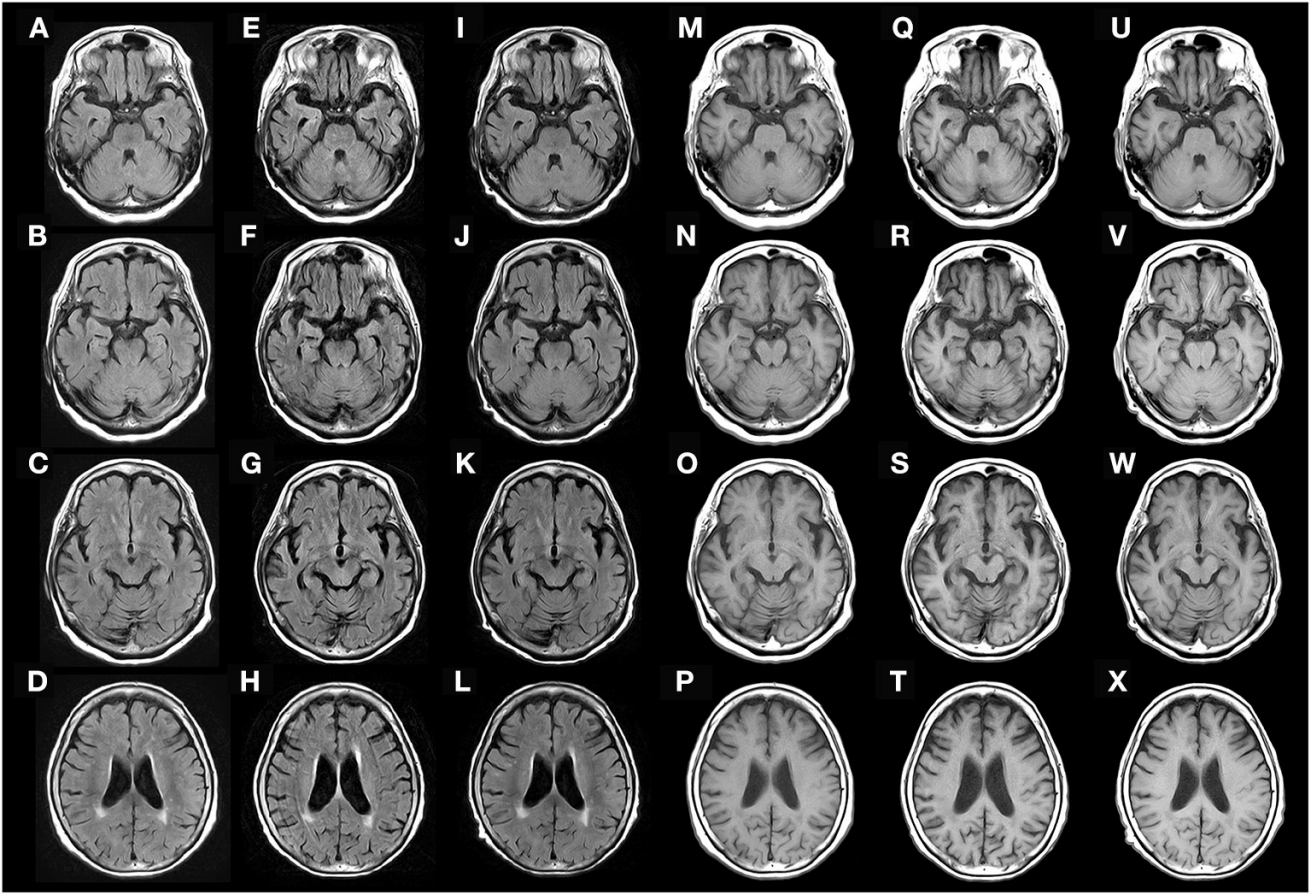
Fluid-attenuated inversion recovery (FLAIR) (A–L) and T1-weighted (M–X) brain MRI obtained 3 months before admission (A–D, M–P), on admission (E–H, Q–T), and 9 weeks after the initial treatment (I–L, U–X). Diffuse cerebral atrophy was observed on admission (E–H, Q–T), but rapidly recovered after immunotherapy (I–L, U–X).
